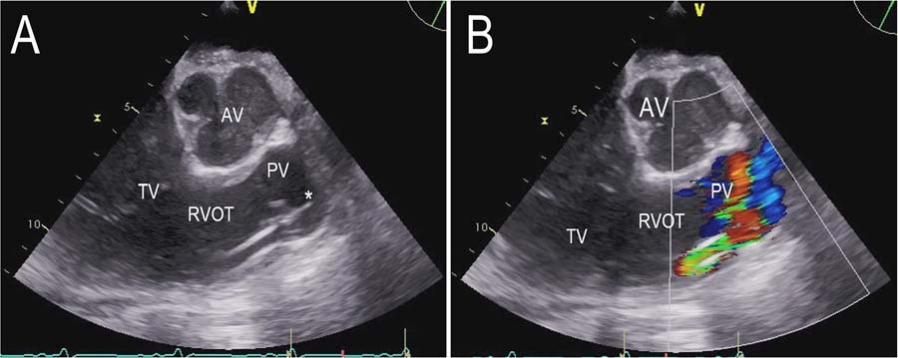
Transthoracic echocardiogram after implantation of a percutaneous RVAD with two cannulas via the right IJV and right FV. Parasternal long-axis view demonstrating the cannula position in the pulmonary valve (A). Color Doppler transthoracic echocardiogram after implantation of a percutaneous RVAD with two cannulas via the right IJV and right FV. Parasternal long-axis view demonstrating severe pulmonary regurgitation due to cannula position in the pulmonary valve (B). AV (aortic valve), PV (pulmonary valve), RVOT (right ventricular outflow tract), TV (tricuspid valve), ^*^ tip of cannula.
